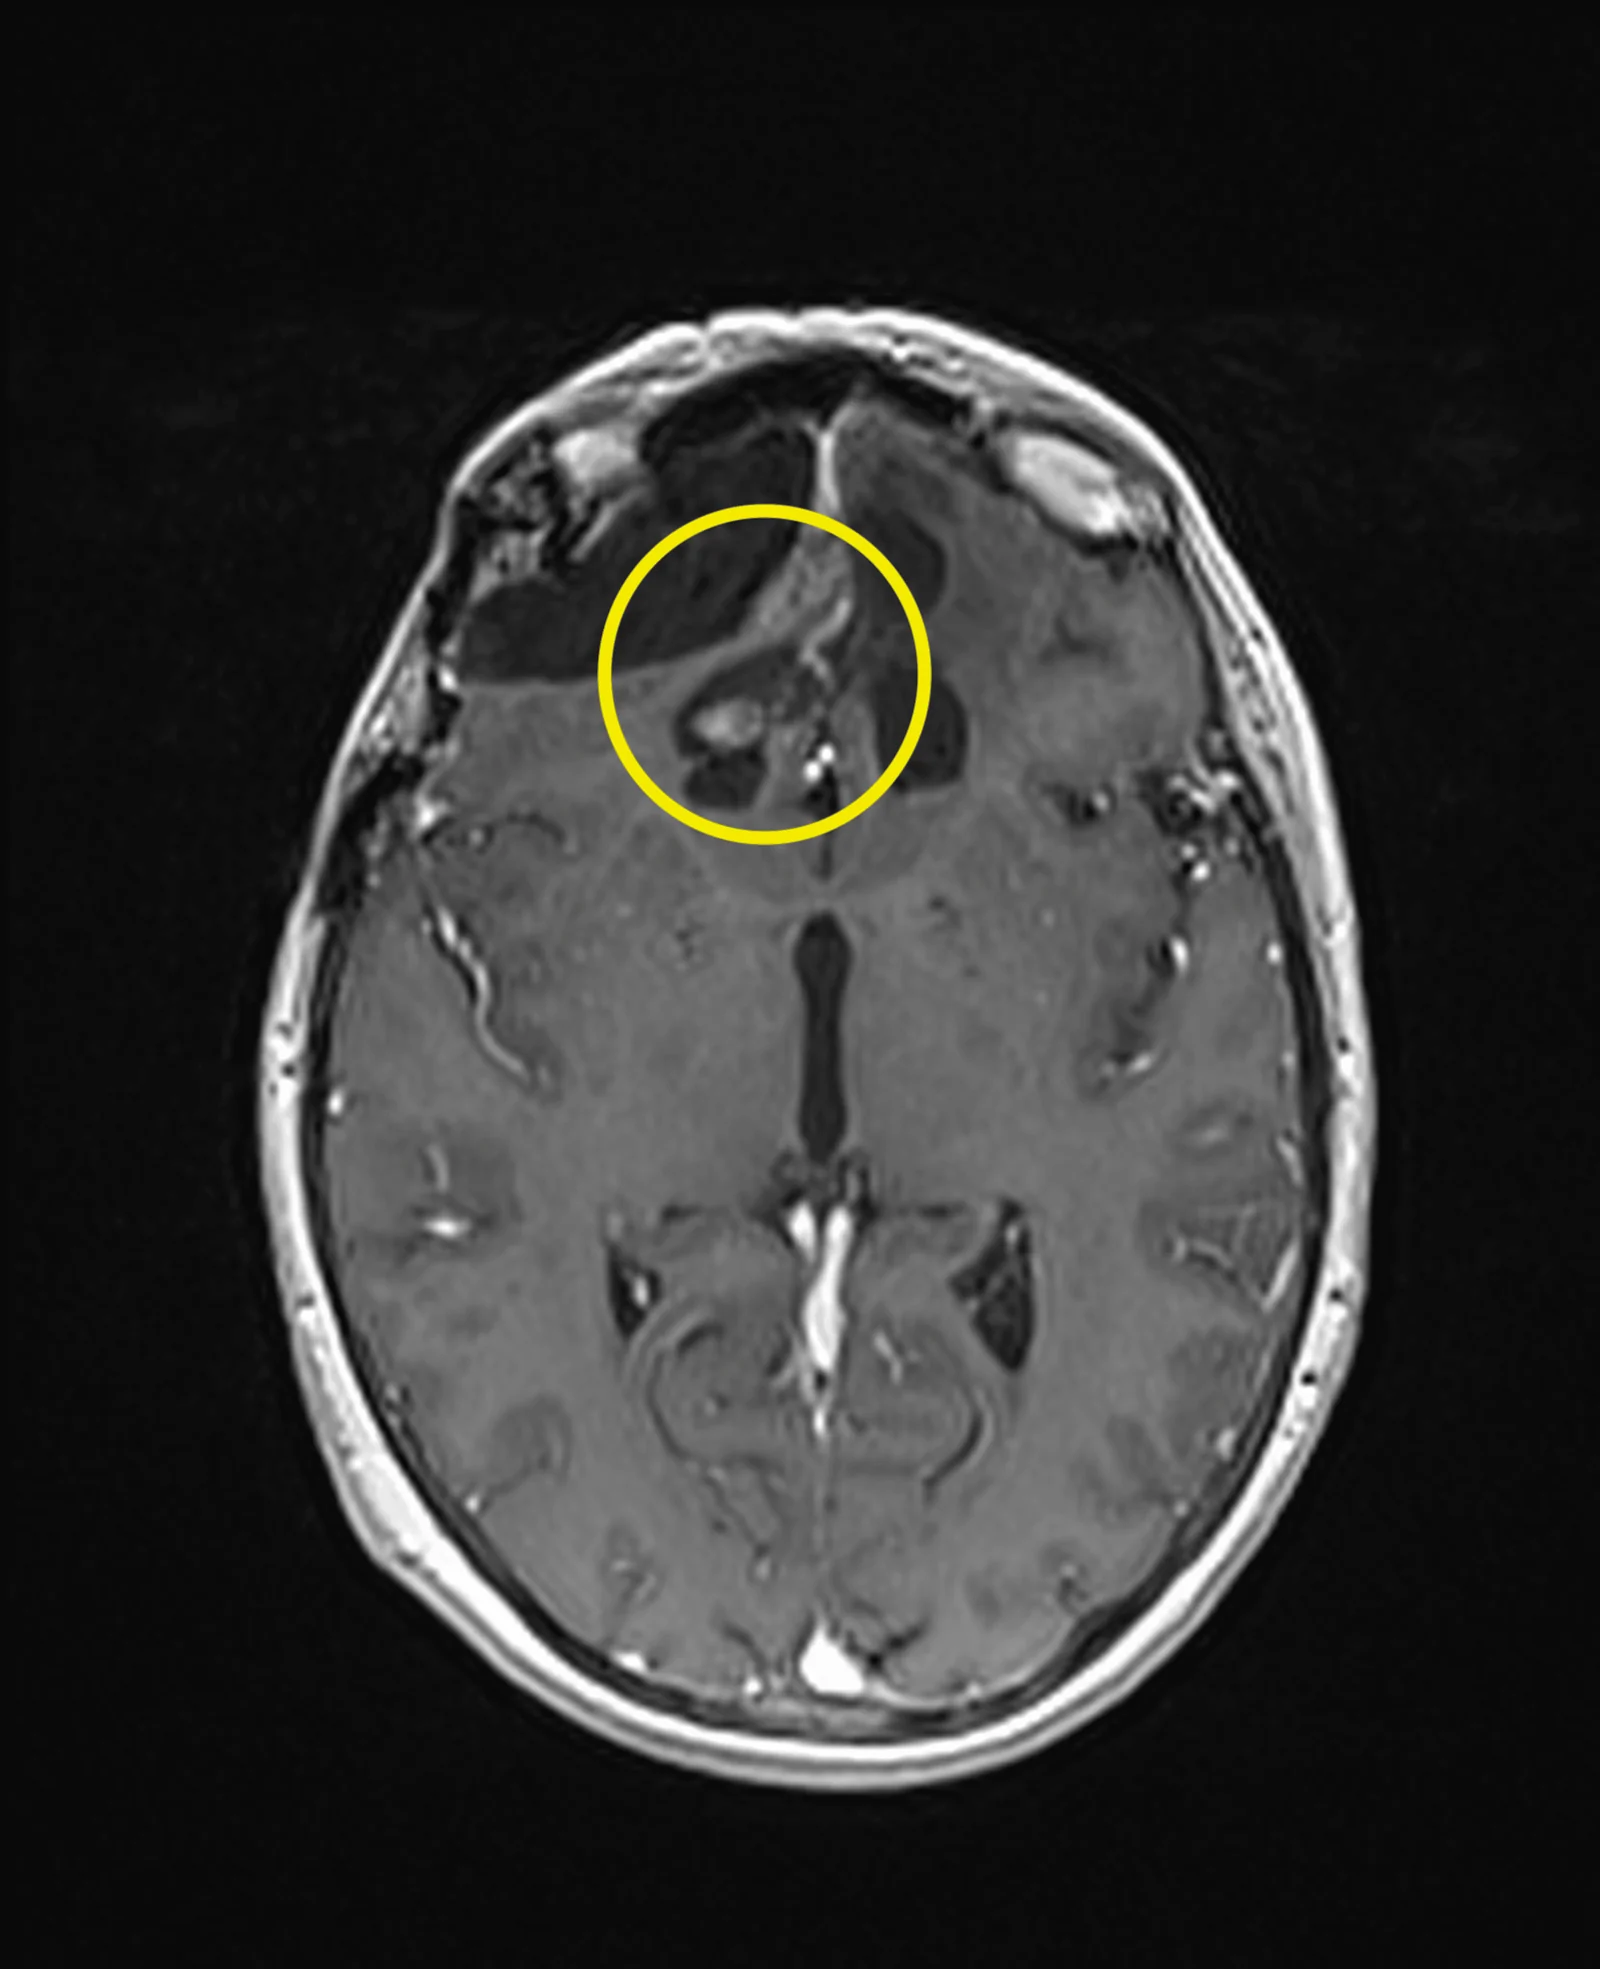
Postoperative post-contrast T1-weighted brain MRI obtained on day 136 Axial post-contrast T1-weighted MRI demonstrating postoperative changes after right fronto-orbital craniotomy. The yellow circle highlights a small left anteroinferior parafalcine residual enhancing lesion, without associated mass effect MRI: magnetic resonance imaging
